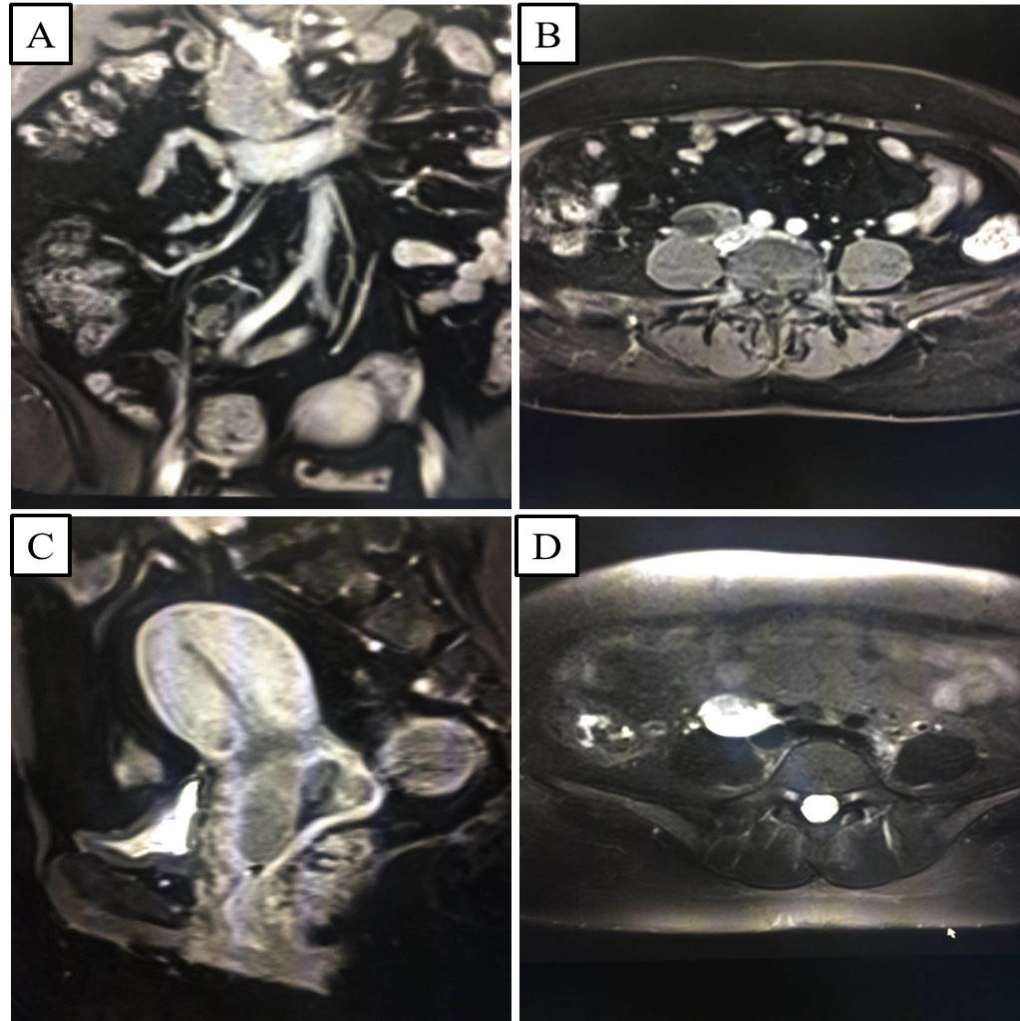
Magnetic resonance imaging (MRI) of cervical cancer and local regional metastatic lymph nodes. A: Coronal view of MRI showing lymph node metastases to the right iliac artery and uneven enhancement. B: Axis view enhancement showing the location of metastatic lymph nodes. C: Sagittal view of cervical cancer. D: Axis view t2-weighted imaging (T2WI) showed the right iliac artery para metastatic lymph node, partial liquefaction and necrosis.
